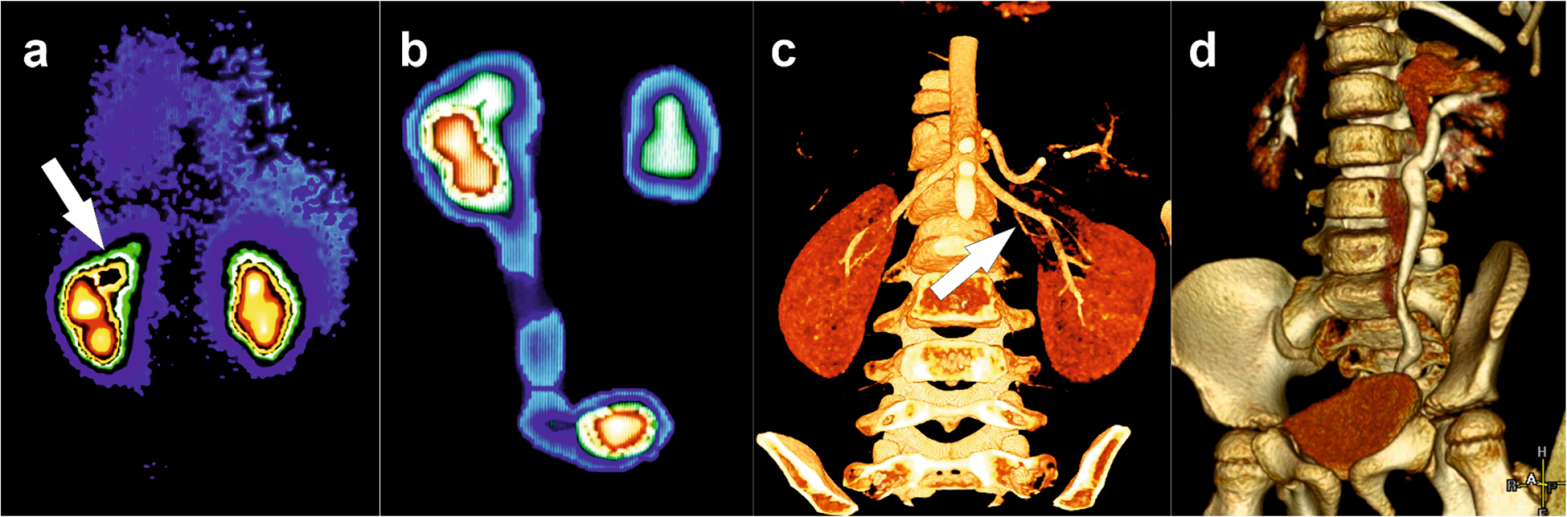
DMSA scans (a and b) show decrease of functioning parenchyma volume and urinary retention in the upper moiety. CT (c and d) show an additional vessel to the upper moiety (arrow), renal triplication and dilation of pelvicalyceal system and ureter of the upper moiety
